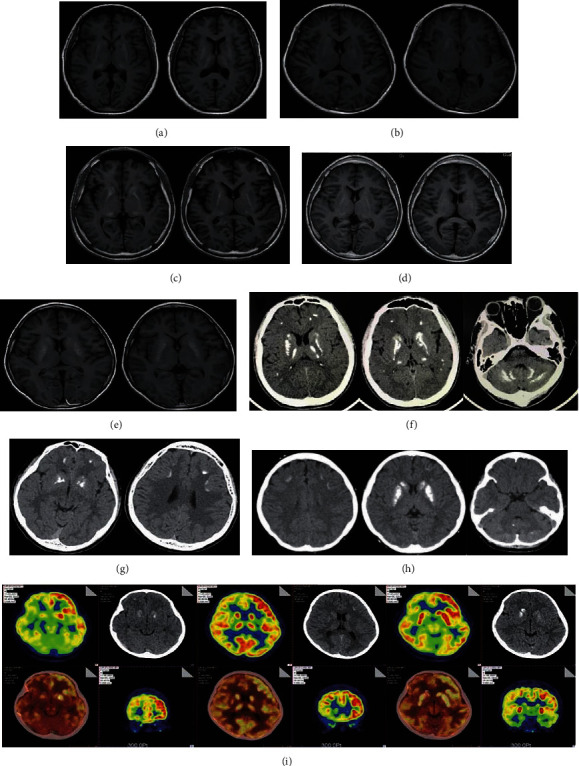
Representative imaging results of all patients. (a)–(e) Representative MR results of cases 1-5. (f)–(h) Representative CT results of cases 3-5. (i) Representative PET-CT results of case 2.
